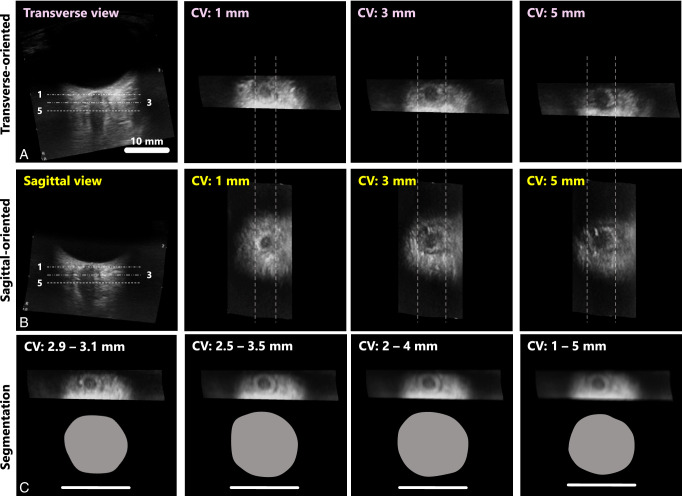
Coronal view (CV) and projection images of the optic nerve sheath. A, Shows coronal slices from a transversely oriented 3D ultrasound scan. B, Shows slices from a sagittally oriented scan. C, Displays mean intensity projection images derived from the transversely oriented scan. In rows (A) and (B), the first column represents the reference viewing plane, followed by coronal views at 1 mm, 3 mm, and 5 mm depths below the optic disc. In row (C), projection images are shown with increasing projection thickness: ±0.1 mm (2.9 to 3.1 mm), ±0.5 mm (2.5 to 3.5 mm), ±1 mm (2 to 4 mm), and ±2 mm (1 to 5 mm).
